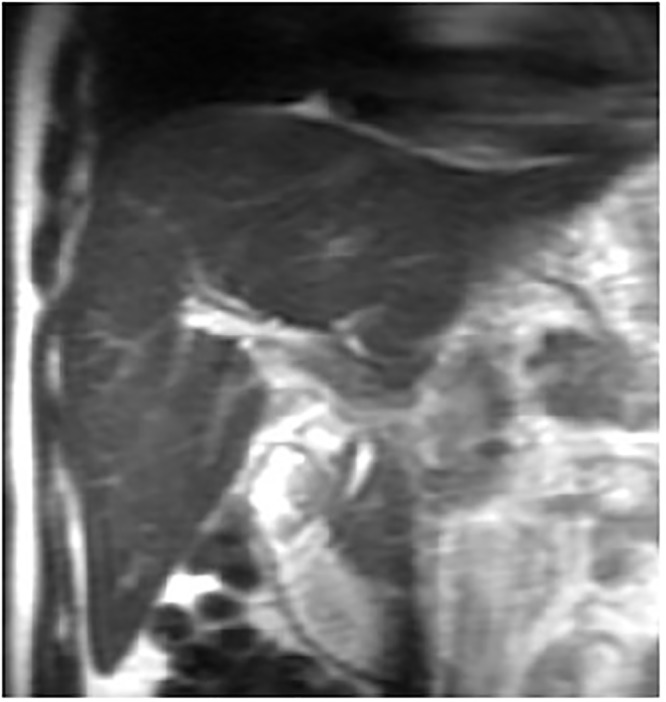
male, 61 years, diffuse hepatomegaly shown on coronal T2-weighted image.
